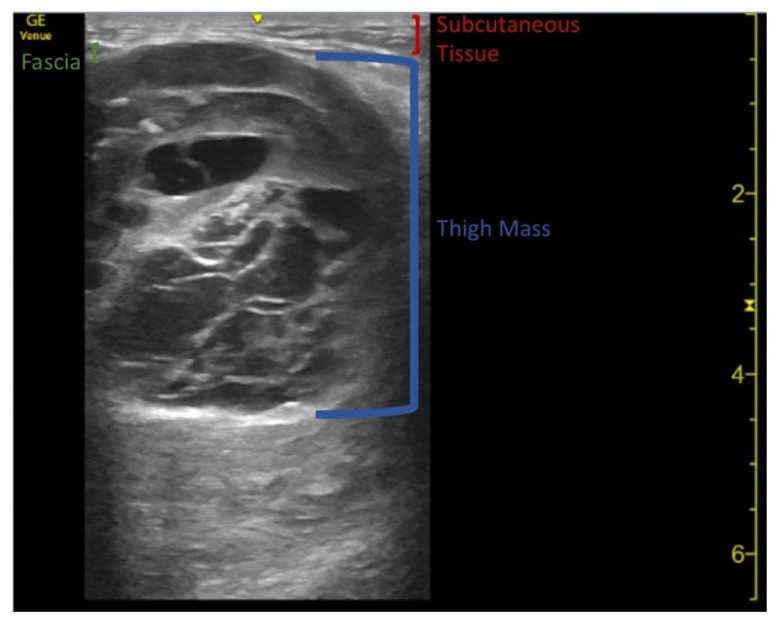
Video Link: https://youtu.be/IVAw2T8e5IY Doppler Video Link: https://youtu.be/XX8m1yv1hrA
